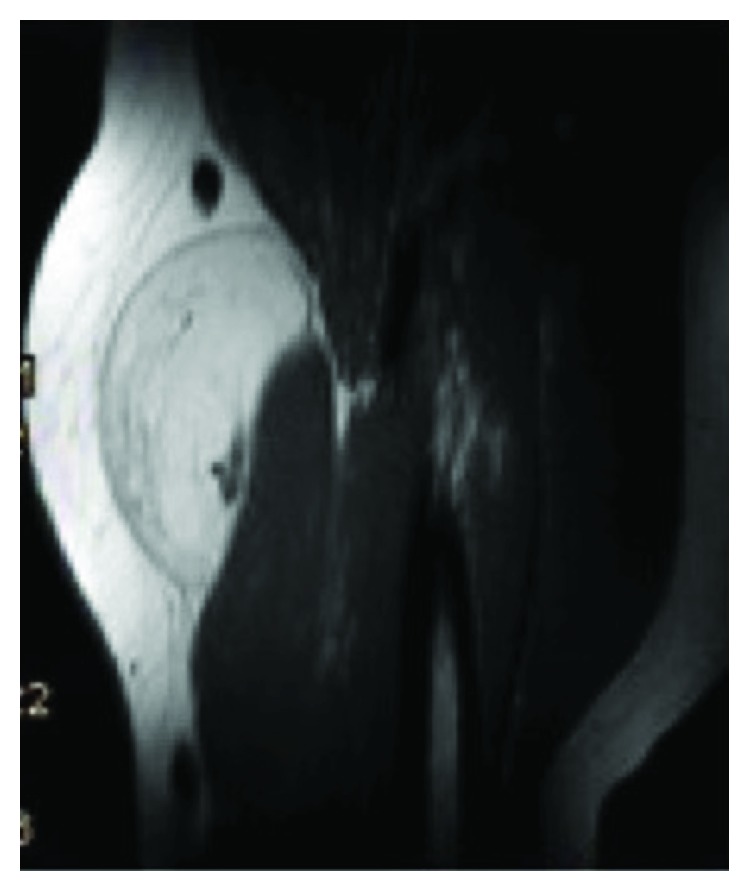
Sagittal MRI image, contrast-enhanced, T1w.
